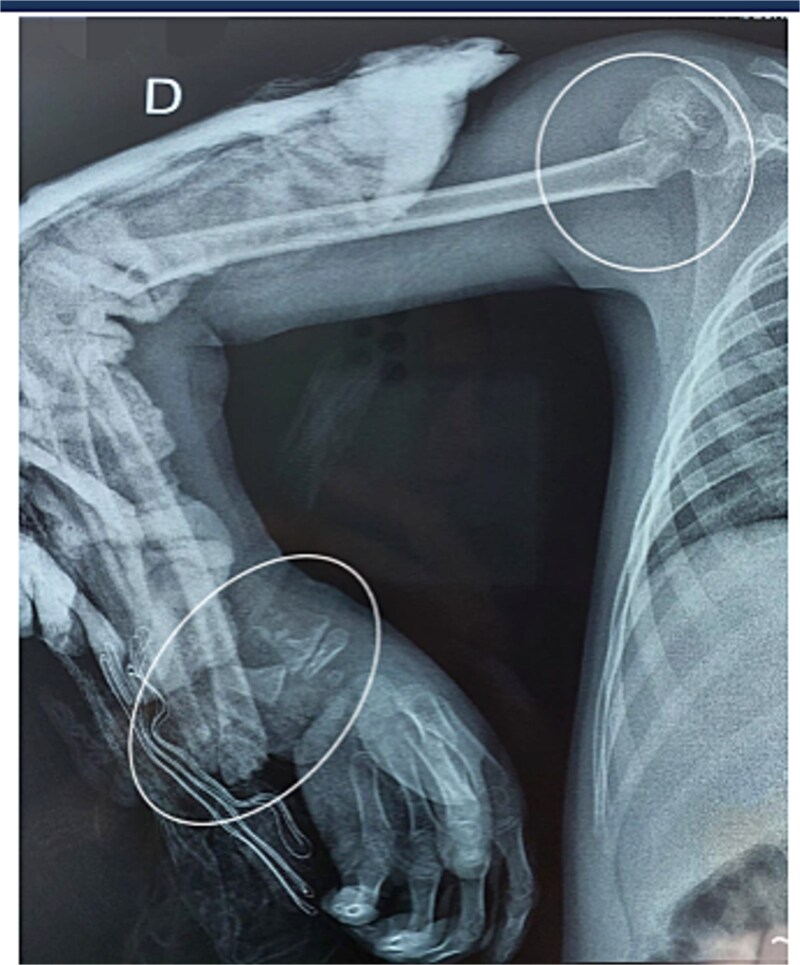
Initial radiograph showing proximal humerus fracture and distal radius and ulna fractures (‘floating elbow’).
